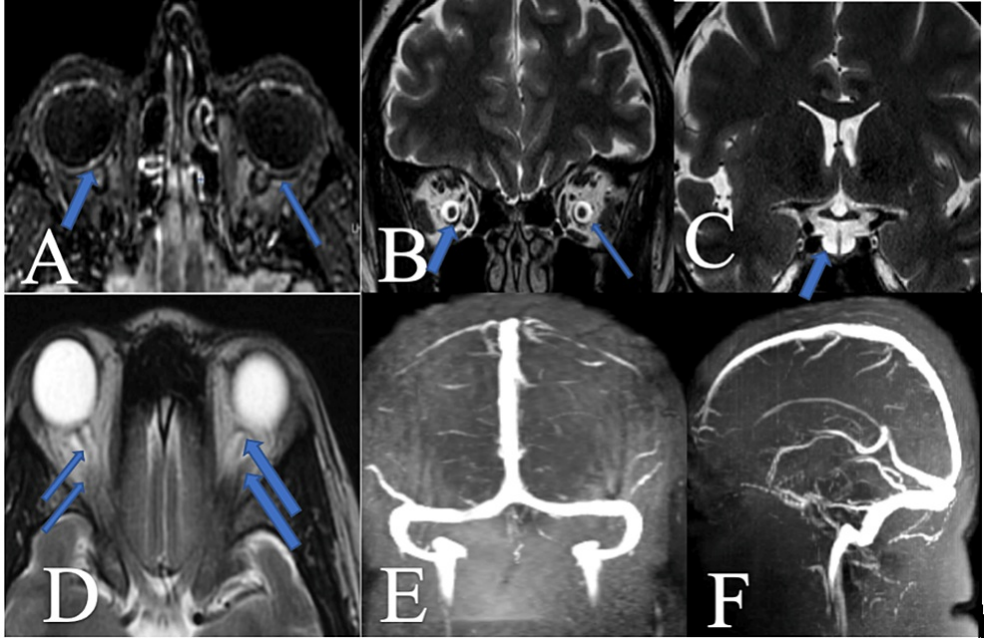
MRI brain and venogram (A) Fluid-attenuated inversion recovery (FLAIR) sequence showed hyperintensity at the optic disc with posterior globe of orbit flattening; (B) T2-weighted (T2W) coronal dilatation of optic nerve sheet; (C) T2-weighted (T2W) coronal dilatation of empty sella turcica; (D) T2W axial orbital cuts showed tortuosity of optic nerve; (E, F) Normal dural venous sinus system
